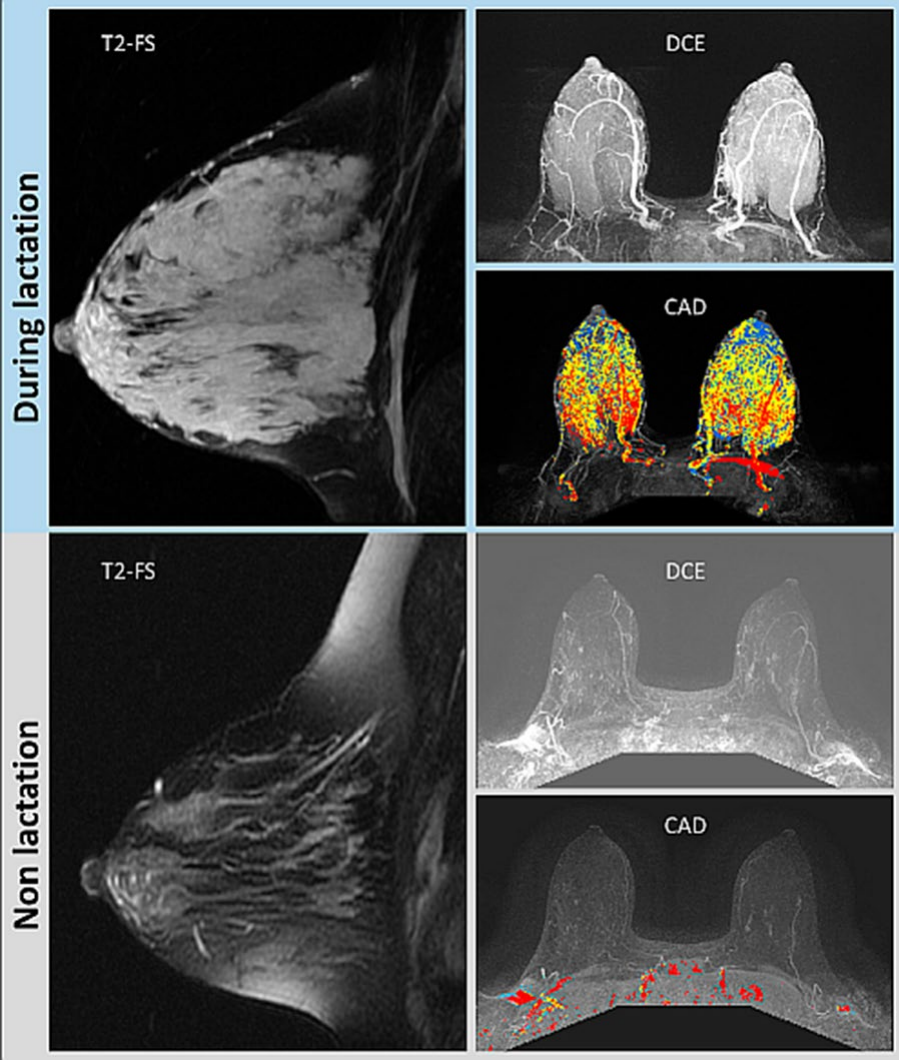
Changes of breast MRI properties during lactation. MR images of a BRCA carrier scanned twice at the age of 37, while lactating for 4 months (upper panel), and 2 years afterwards, post-weaning (lower panel) are presented. The sequential examinations demonstrate the marked changes in the breast composition and vascular properties between the two periods. A rich fibroglandular tissue, which is exhibited during lactation, reduces dramatically post weaning. Respectively, the breast perfusion reduces considerably, from BPE 3 to BPE 1, as shown on DCE subtraction MIP images, as well as on DCE CAD maps. Add DCE MIP
